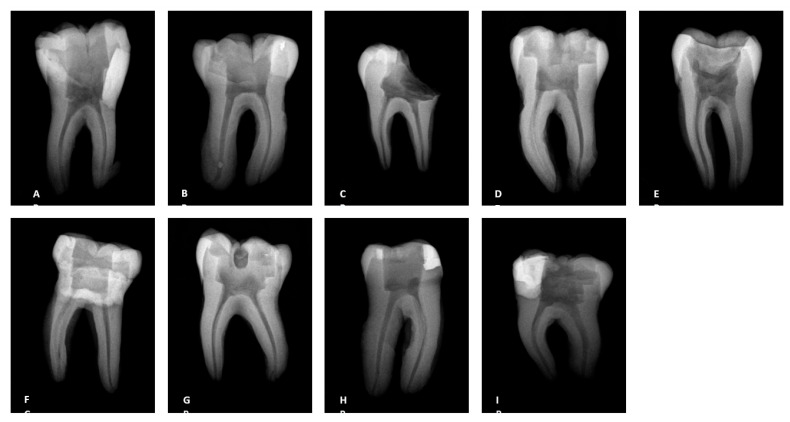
Radiographs of the teeth treated with three different irrigation protocols. (A–C) Laser #1 (NM), (D–F) mid-root laser #2 (CA), and (G–I) UAI.
